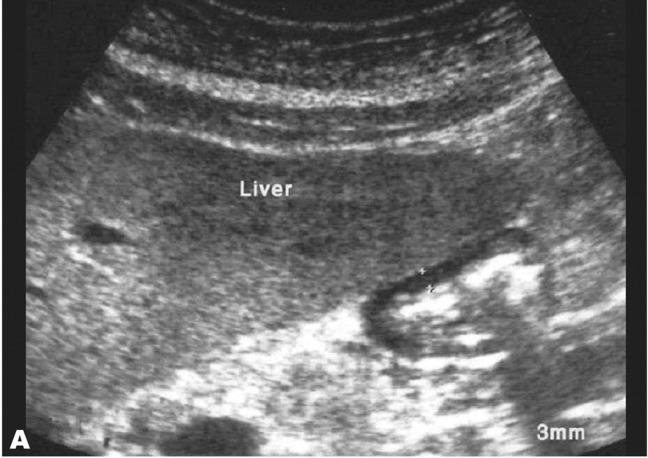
Transverse abdominal ultrasound image in a healthy 38-year-old woman demonstrating normal hypoechoic gastric wall thickness of less than 5 mm.
